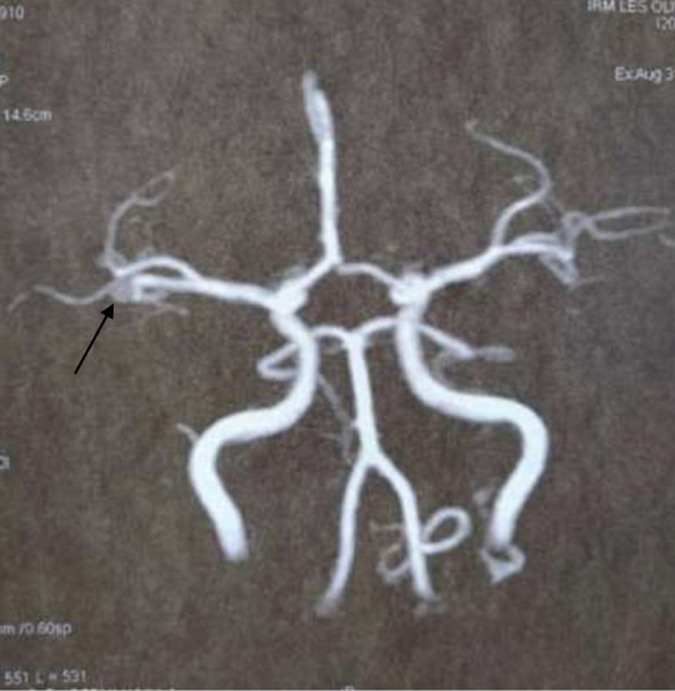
angio-MRI sequences showing a hyper vascular lesion
